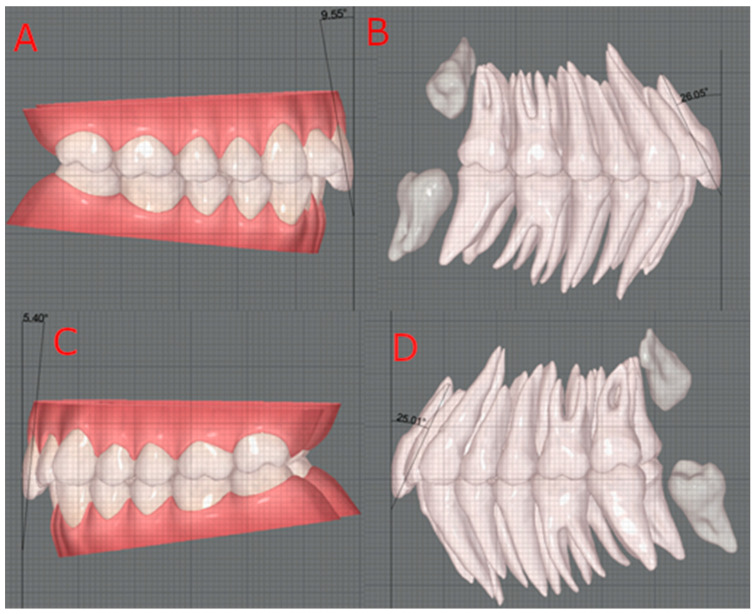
Torque measurement: right upper incisor without Cone Beam Computed Tomography (CBCT) (A); right upper incisor with CBCT (B); left upper incisor without CBCT (C); left upper incisor with CBCT (D).
